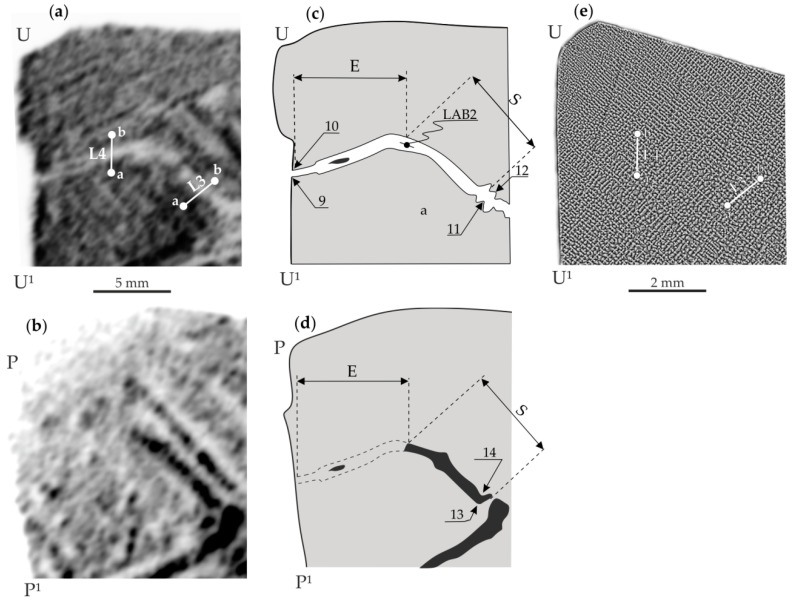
Examples of X-ray topograms obtained from fragment B of surface S_1_ and fragment B* of surface S_2_ (a,b) with their schemes (c,d) and the dendritic structure visualised on the surface S_1_ (e). Since the topogram from fragment B* was obtained from the mirror surface of fragment B, it was transformed by mirroring for easier comparison of areas of the same LAB visualised on both surfaces S_1_ and S_2_.
